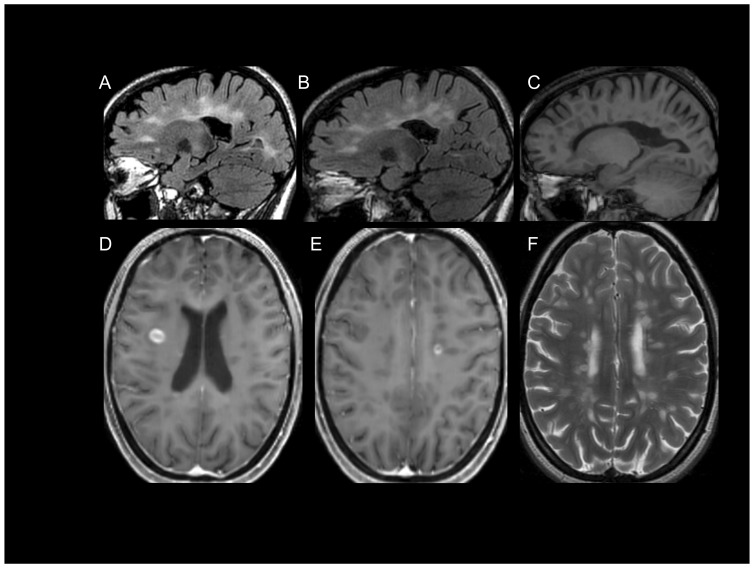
Brain MRI of patient 73F. (A) FLAIR image from September 2011 showing characteristic lesions in a parasagittal plane. (B–F) MRI from May 2012 revealing characteristic lesions on FLAIR imaging as before (B), T1 hypointensities (C), characteristic contrast enhancing lesions post IV Gadolinium (D, E), and characteristic lesions on T2 weighted axial image (F).
